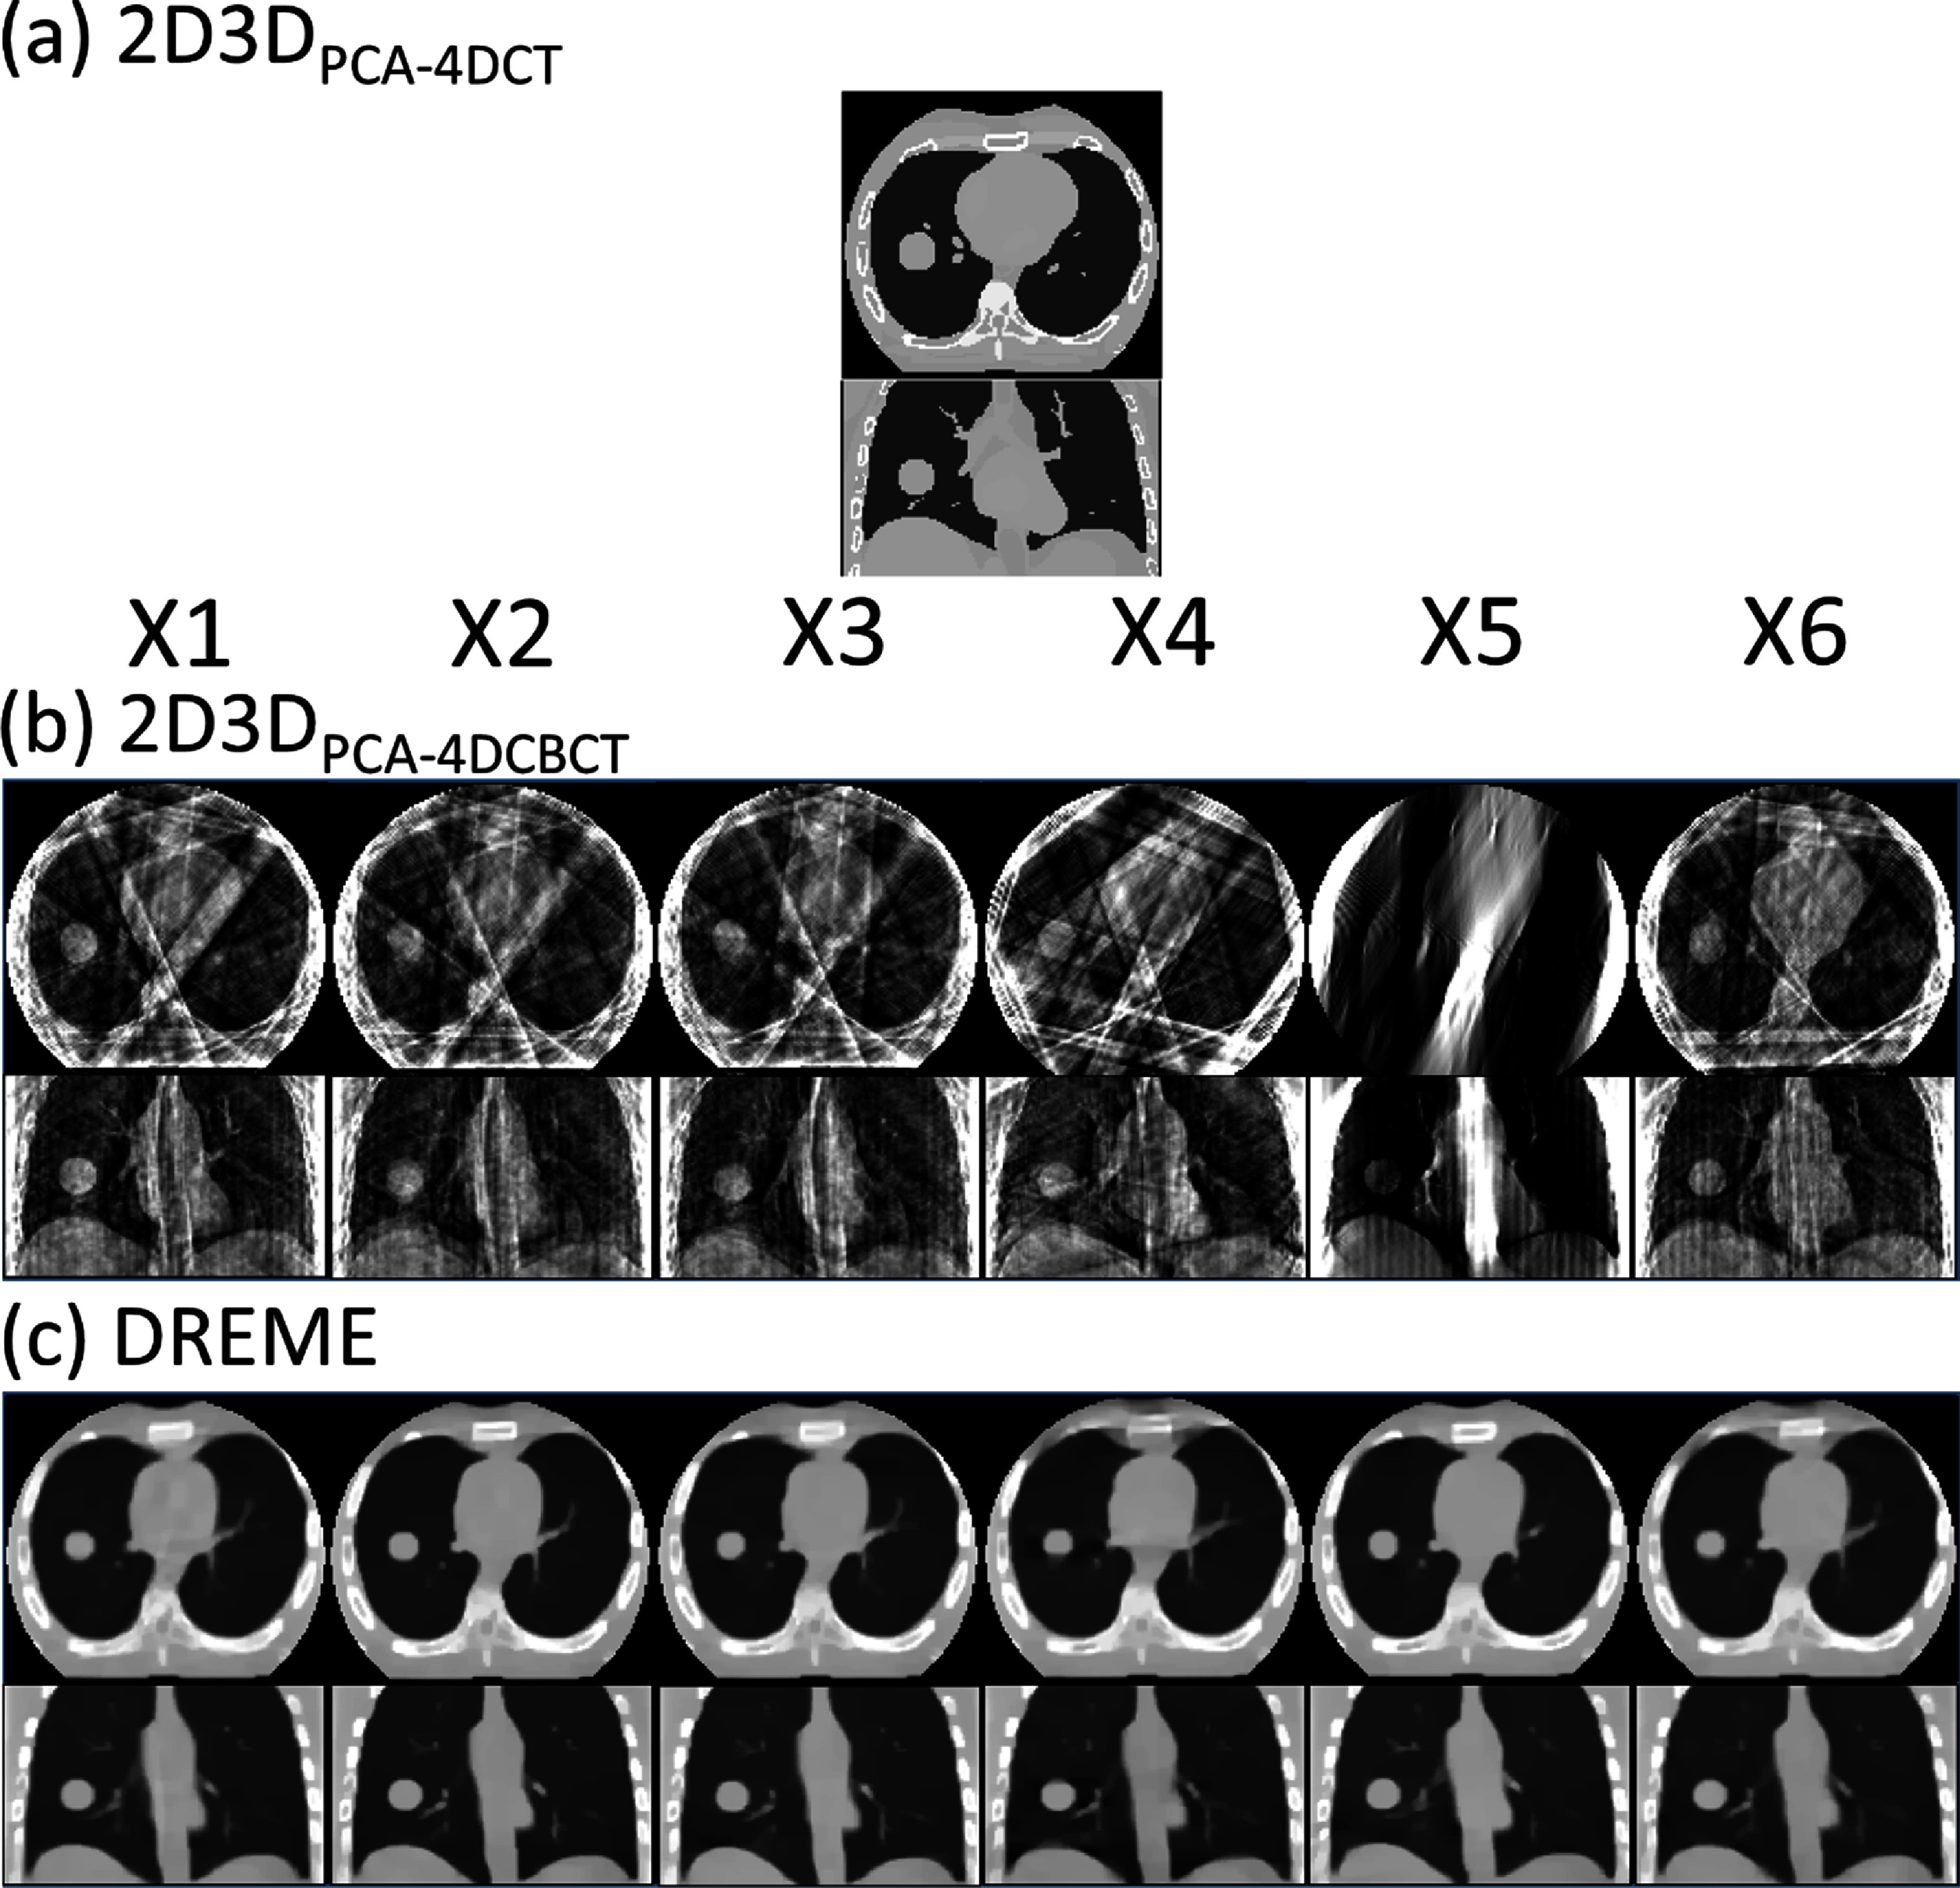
Comparison of reconstructed reference CBCTs in the comparison study. 2D3D_PCA-4DCT_ assumed the existence of an artifact-free pre-treatment 4D-CT scan and used the end-of-exhale phase as the reference anatomy. 2D3D_PCA-4DCBCT_ and DREME reconstructed the reference CBCTs using the pre-treatment scans, thus the anatomy is up-to-date and specific to daily motion scenarios. The reference CBCTs of 2D3D_PCA-4DCT_ were reconstructed from the end-of-exhale phase after projection phase sorting, suffering from significant under-sampling and motion-related artifacts. DREME reconstructed the reference CBCTs with simultaneous motion estimation/compensation, resulting in minimal motion-related artifacts.
